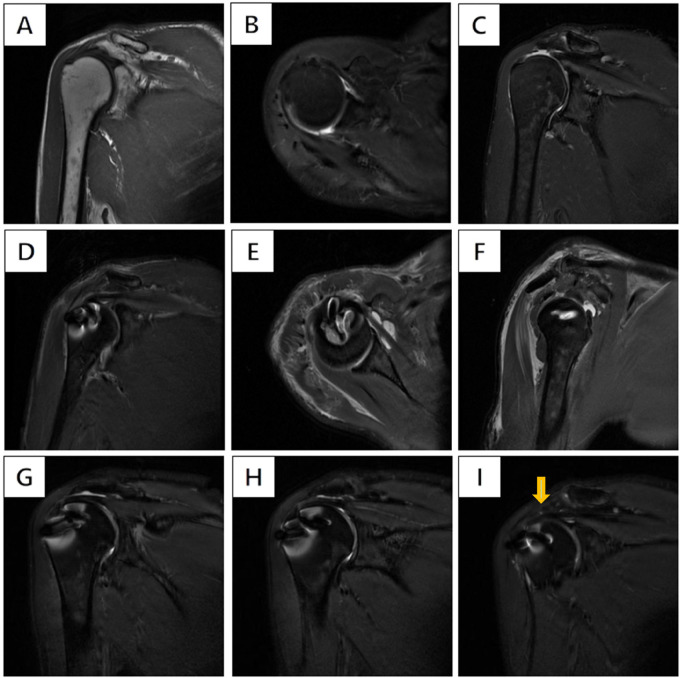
Imaging shows a case repaired using modified Kessler tension-reducing suturing combined with the double-row technique. (A–C) Preoperative MRI revealing a full-thickness tear with tendon retraction. (D–F) Early postoperative MRI showing successful tendon reduction and fixation. (G–I) At the 1-year postoperative follow-up, MRI demonstrated good healing of the torn tendon edge, with no evidence of muscle atrophy and satisfactory tendon tension.
